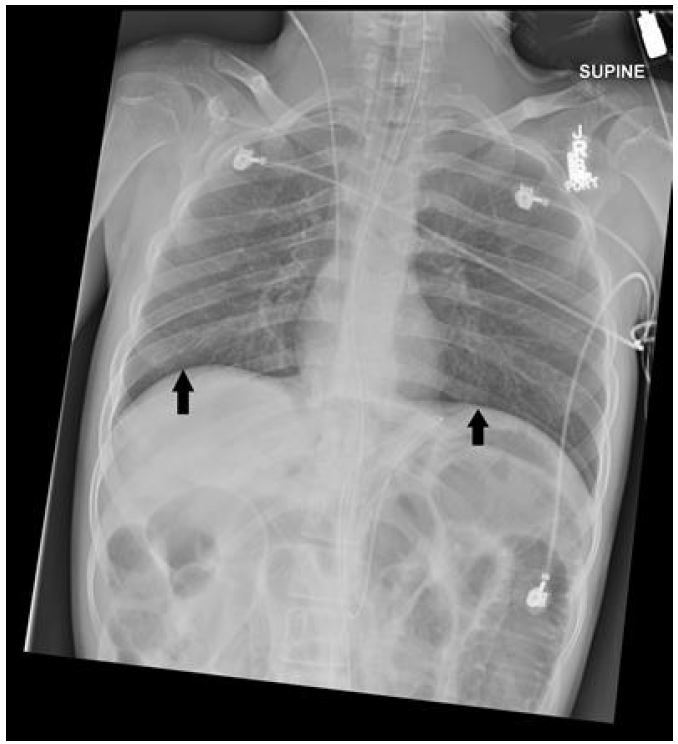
Chest x-ray performed after needle decompression of abdomen demonstrating improved diaphragmatic excursion (black arrows).
